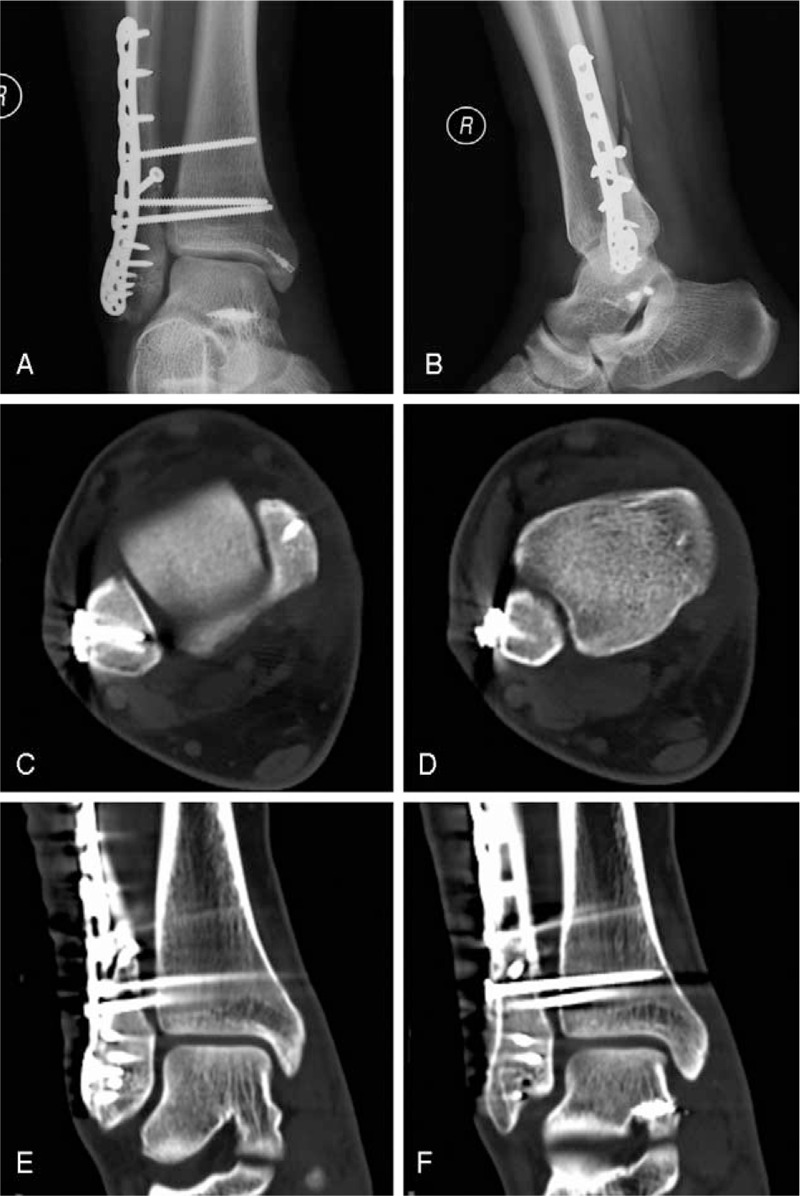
(A,B) Anteroposterior and lateral X-ray image re-operation. (C–F) post-operative CT views shows the syndesmosis and mortise almost normal.
